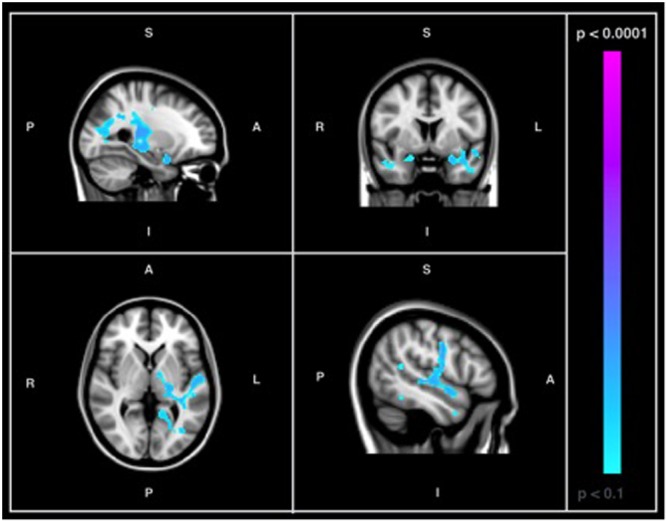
Images showing GM and WM regions where SD_BOLD_ is negatively associated with ADNI-MEM scores in healthy controls (*p* < 0.1, corrected for multiple comparisons). Images on overlaid on T1-weighted MNI152_T1_2mm standard template provided by the Functional MRI of the Brain’s Software Library.
